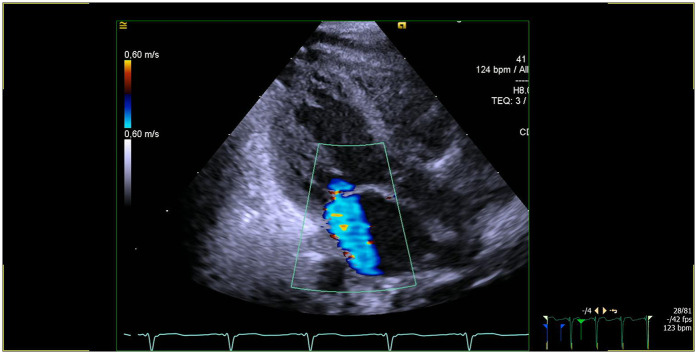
Transthoracic echocardiography, apical long axis view, of normal right ventricular contractility and severely impaired left ventricular contractility associated with moderate mitral regurgitation.
